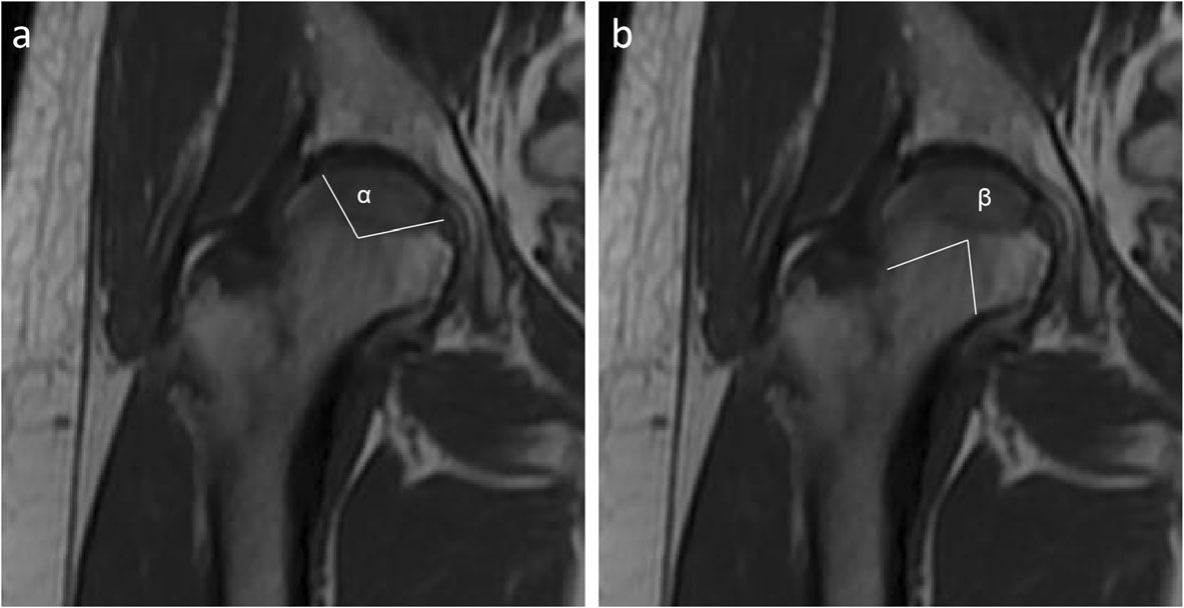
a The angle α was defined as the angle corresponding to the necrotic site on an MRI image. b The angle β was defined as the central angle corresponding to the femoral head in the same image (the angle between the connecting lines from the center of the femoral head to the femoral head-neck junction)
